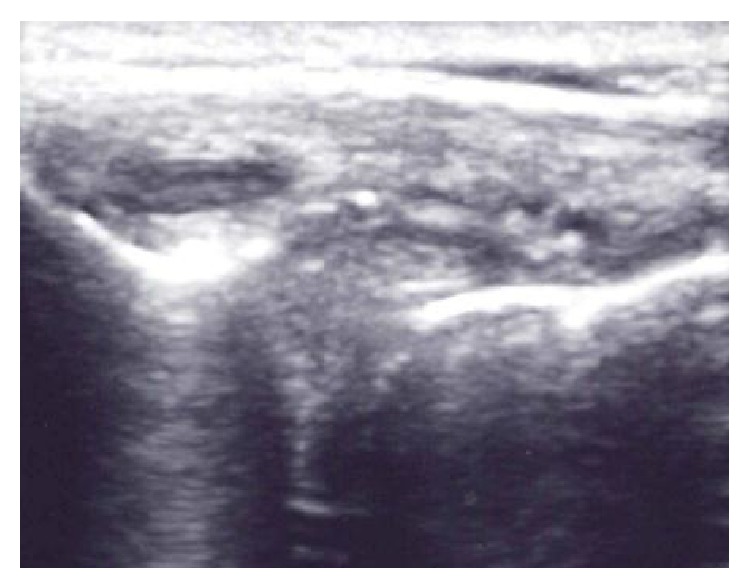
Ultrasound image in the coronal plane demonstrating resolution of the cyst immediately after aspiration and injection.
